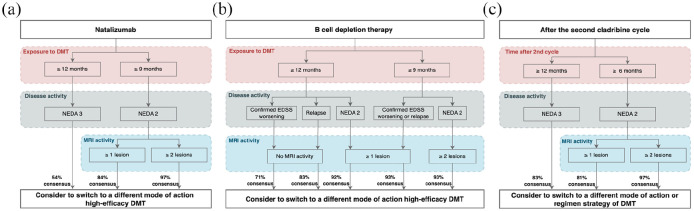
Horizontal switch algorithms in patients with high sNfL (>90th percentile): (a) From natalizumab; (b) From B-cell depletion therapy; and (c) From cladribine to different mode of action of DMT. Example of algorithm: If your patient is currently receiving B-cell depletion therapy for at least 12 months and has high sNfL (>90th percentile) jointly consider with your patient to switch to a different mode of action HET if your patient has relapses but no MRI activity. EDSS: Expanded Disability Status Scale; DMT: Disease-Modifying Therapy; MRI: magnetic resonance imaging; NEDA: No evidence of disease activity was defined as NEDA2, i.e., no relapses and no EDSS worsening or as NEDA3 no relapses, no EDSS worsening and no MRI activity.
